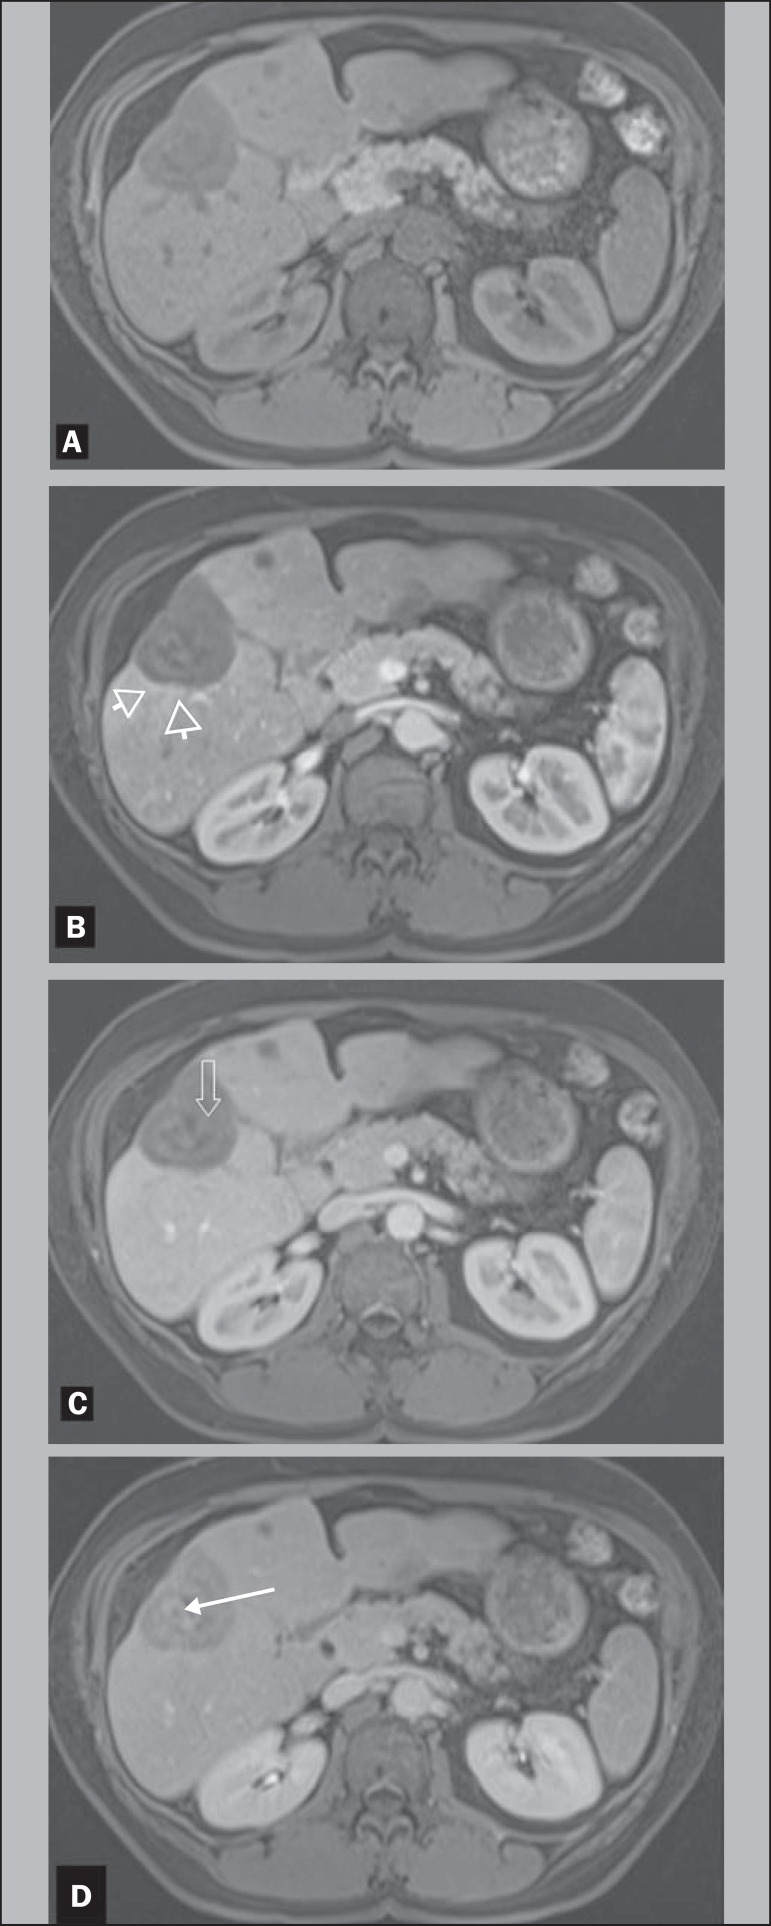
A 42-year-old female with HEHE. Axial, T1-weighted pre and postcontrast
